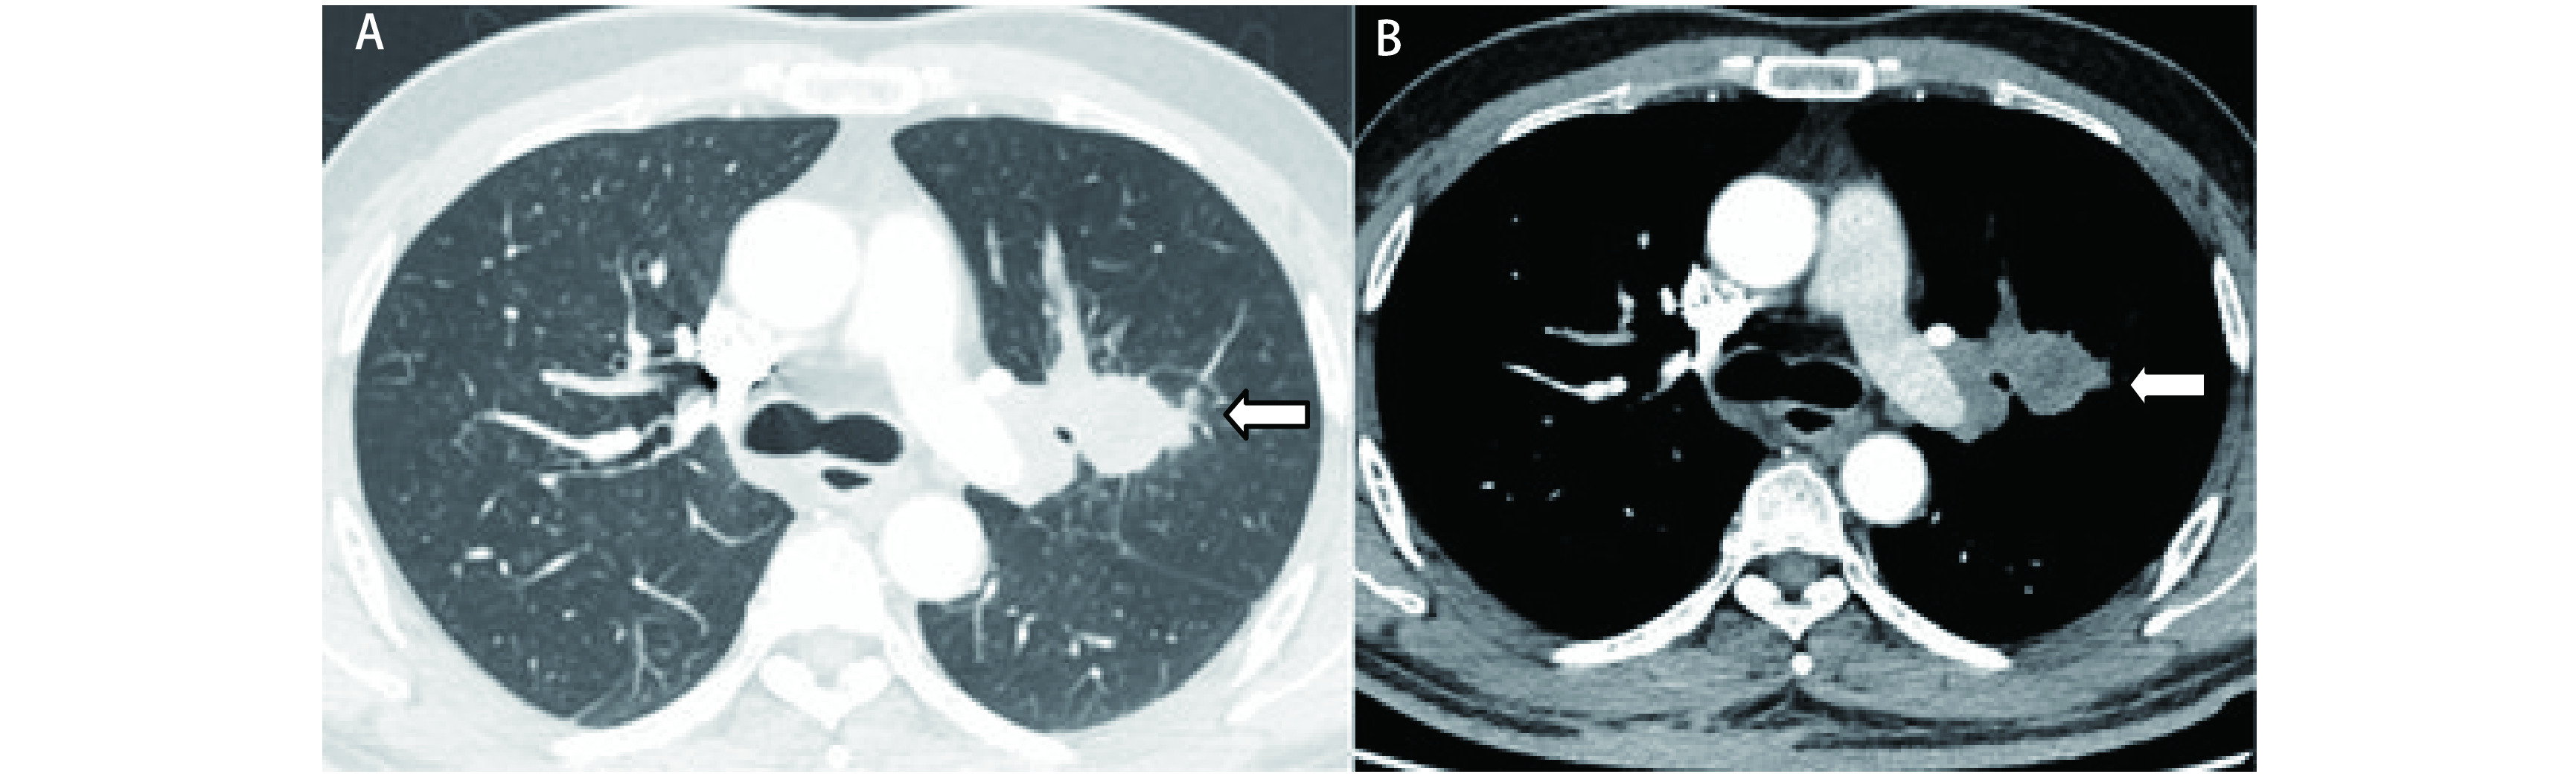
胸部增强CT检查。A：肺窗, 左肺上叶中央型肺癌, 肿瘤包绕肺动脉干(白色箭头所示); B：纵隔窗, 肿瘤呈分叶状, 包绕肺动脉干, 肿瘤43 mm×37 mm×25 mm(白色箭头所示)。 Thoracic enhanced CT scanning. A: pulmonary window: The tumor is located in the center of the left upper lobe (as indicated by the white arrow); B: mediastinal window: The tumor was lobulated and invades the the left pulmonary artery (as indicated by the white arrow). The tumor size is 43 mm×37 mm×25 mm.
